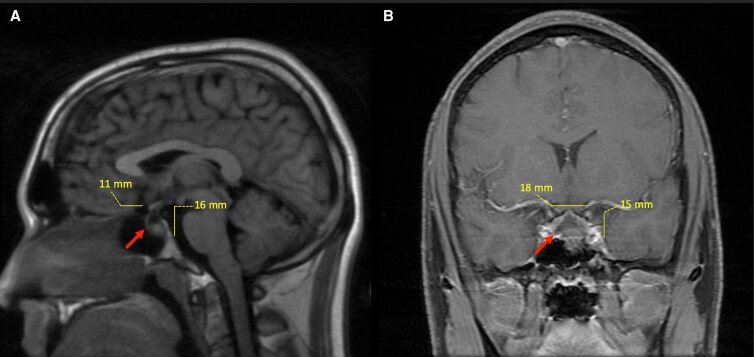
Initial magnetic resonance imaging of the brain with and without IV contrast. (A) Sagittal T1 flair view. (B) Coronal with contrast and fat-saturated view. There is heterogenous composition of the Rathke cleft cyst (identified by arrows in Fig. 1A). The cyst does have a significant size of 1.1 × 1.8 × 1.5 cm in the anteroposterior, transverse, and craniocaudal dimensions, but it does not cause mass effect (Fig. 1B).
